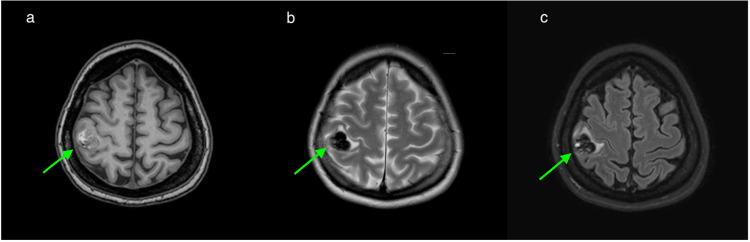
T1WI, T2WI and FLAIR images The lesion is heterogeneously hyperintense on T1WI (a). On T2WI (b) and FLAIR (c) images, the lesion is hypointense except for a high signal component in the superolateral part of the lesion. Perilesional edema is seen on T2WI and FLAIR images. T1WI : T1-weighted images; T2WI: T2-weighted images; FLAIR: fluid-attenuated inversion recovery.
